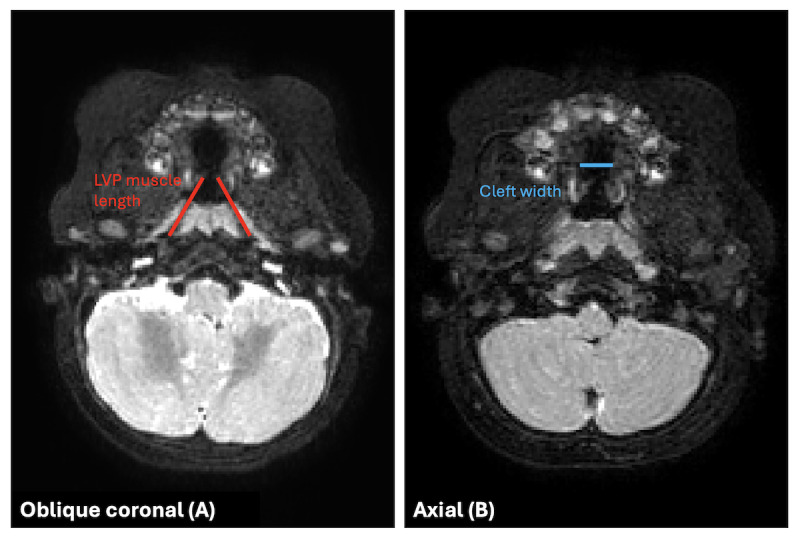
Presurgical magnetic resonance image of an infant labeled with cleft palate with cleft width (A) and levator veli palatini (LVP) muscle length (B).
